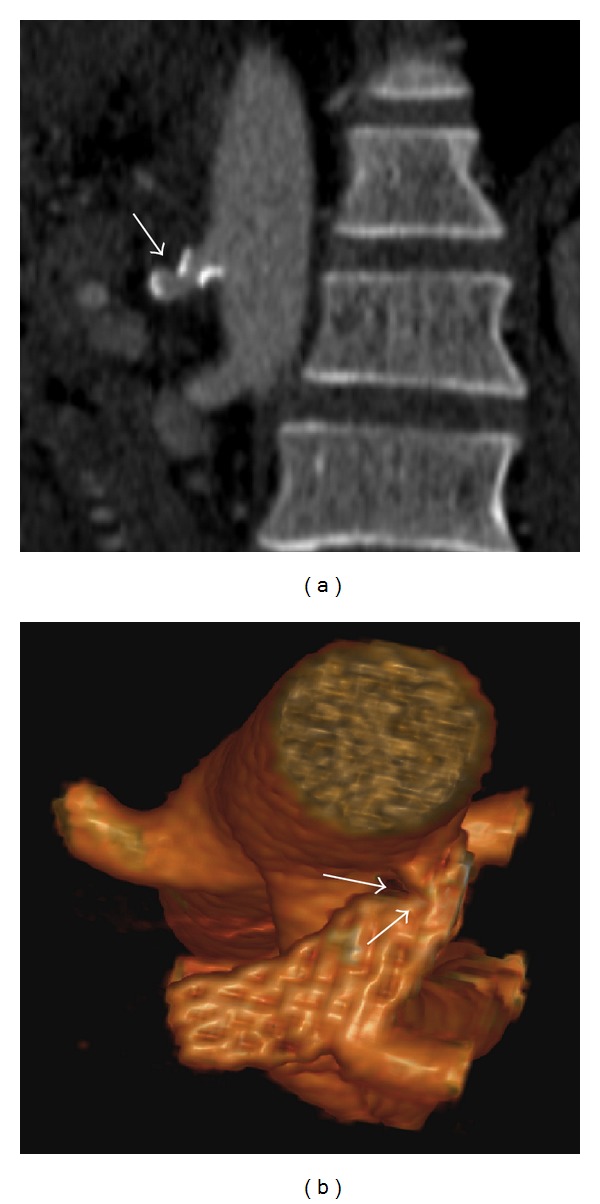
Sagittal view (a) and 3D reconstruction (b) of the CTA at 15-month follow up show stent patency and focal residual stenosis (arrows) at proximal celiac artery.
